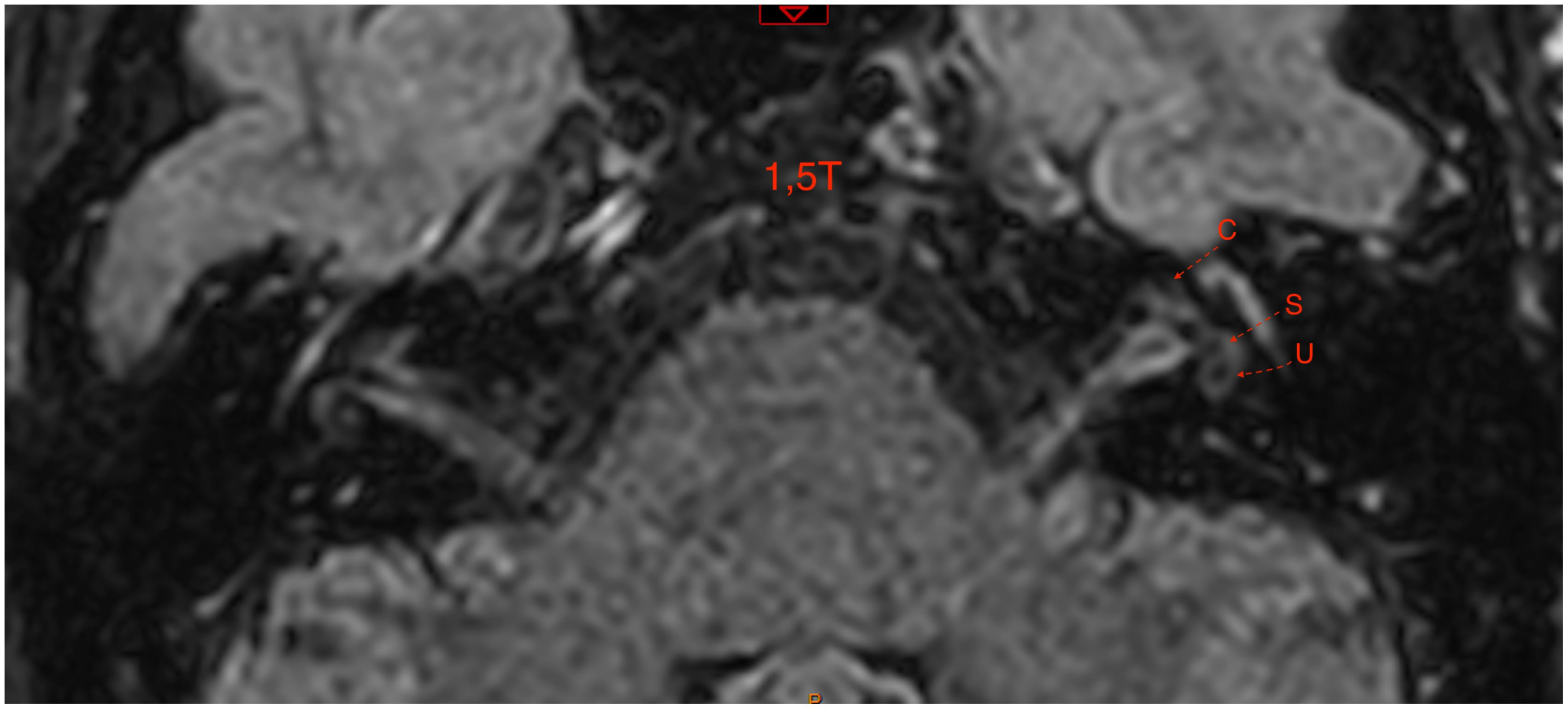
1.5 T MRI axial scan, sequence 3D-FLAIR, left ear with grade 1 of Barath in cochlea and vestibule.
